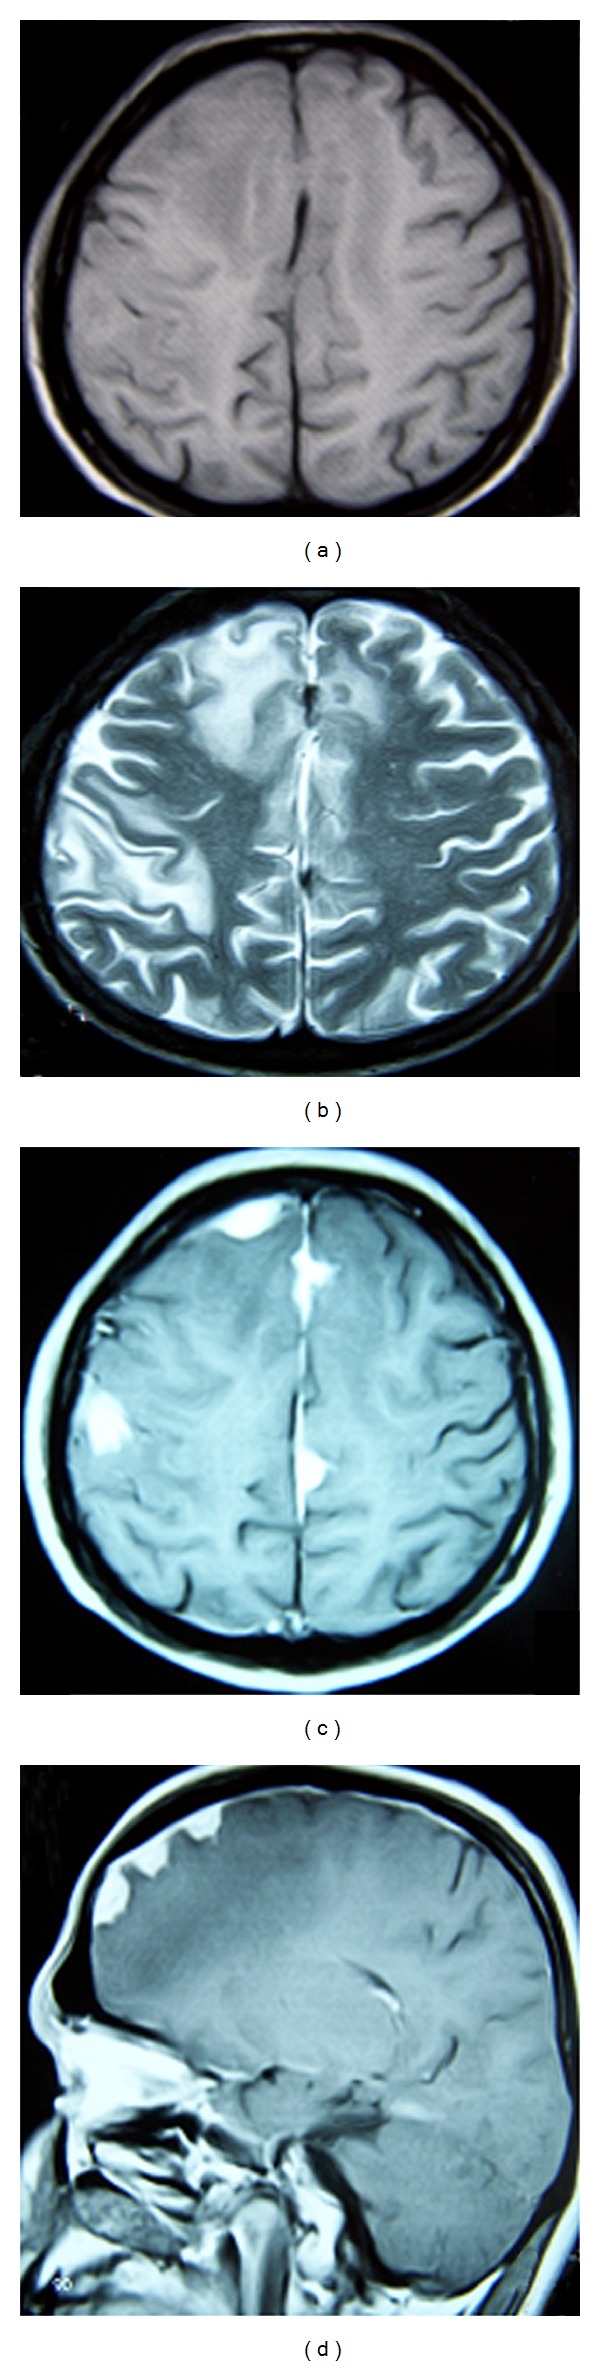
Cranial magnetic resonance imaging (MRI) shows multiple dural-based enhancing lesions on unenhanced (a) and enhanced T1-weighted axial (c) and sagittal (d) images with cerebral edema on T2-weighted images (b).
